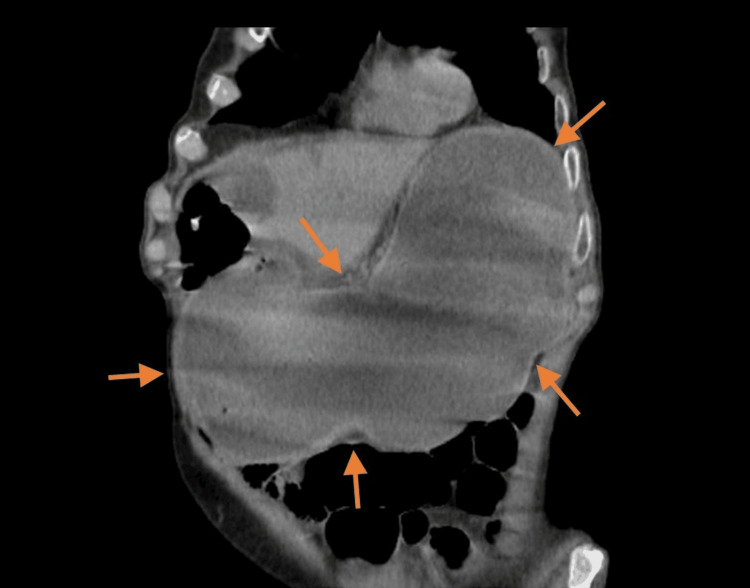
An abdominal CT scan shows gastric dilatation (arrows).
